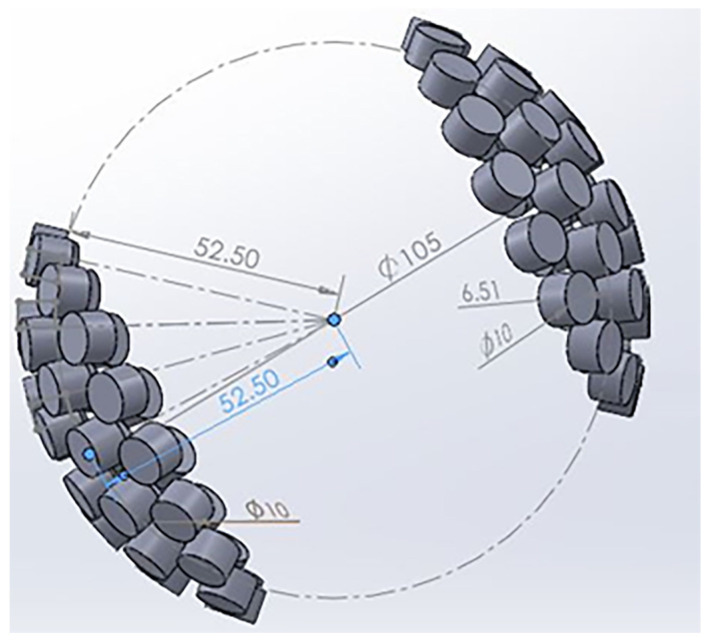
Final CAD design for ultrasonic field generation.
